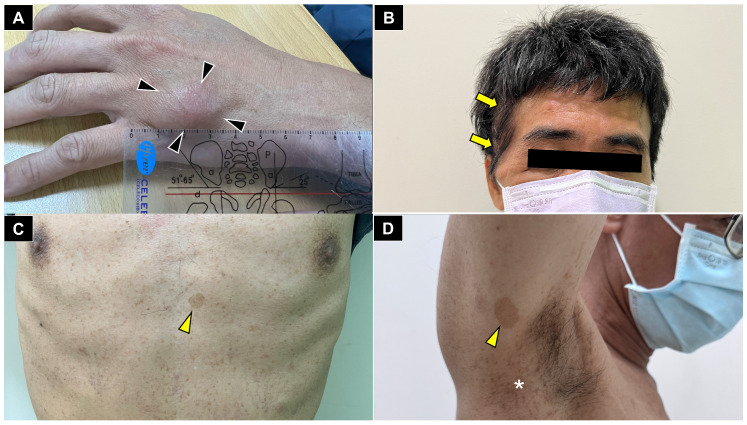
A 45-year-old male presenting with concurrent cutaneous and skeletal manifestations of neurofibromatosis type 1 (NF1). (A) Clinical photograph demonstrating a firm, ovoid subcutaneous mass (black arrowheads) located between the fourth and fifth metacarpals, approximately 3.5 cm in size. The patient reported a three-year history of gradual enlargement with recent onset of perilesional paresthesia. (B) Oblique craniofacial view demonstrating a visible bony contour depression over the right temporal region (yellow arrows). In the context of a childhood history of ophthalmologic surgery, this asymmetry raises suspicion for NF1-associated sphenoid wing dysplasia, a recognized osseous criterion under the revised diagnostic framework [1,2]. (C) Anterior trunk photograph demonstrating multiple hyperpigmented macules, including a representative café-au-lait macule exceeding 15 mm in greatest diameter (yellow arrowhead). (D) Right axillary region demonstrating a café-au-lait macule (yellow arrowhead) and intertriginous freckling consistent with the Crowe sign (white asterisk) [1,2]. Collectively, this patient fulfilled the 2021 revised international consensus criteria for NF1 [1] on the basis of multiple independent features: (1) six or more café-au-lait macules, (2) intertriginous freckling, (3) characteristic osseous involvement consistent with sphenoid wing dysplasia, and (4) a first-degree relative with confirmed diagnosis of NF1. Although molecular genetic confirmation was unavailable due to institutional resource limitations, the constellation of clinical findings was sufficient to establish a definitive clinical diagnosis of NF1 [1,2]. Preoperative brain MRI further excluded intracranial neurofibromas and optic pathway gliomas, addressing the right temporal depression and ophthalmic history. This case underscores the importance of systematic whole-body inspection when evaluating an apparently isolated soft tissue mass of the hand.
